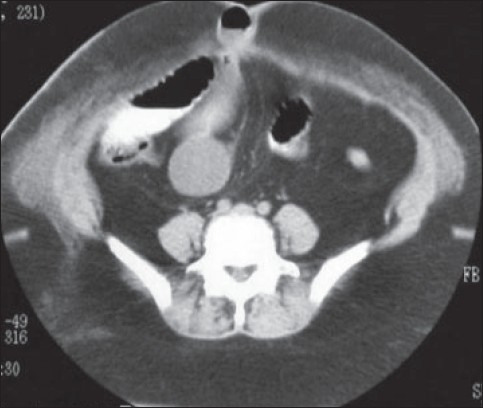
CT scan abdomen showing the complete obstruction of the herniated small bowel loop in the port site
